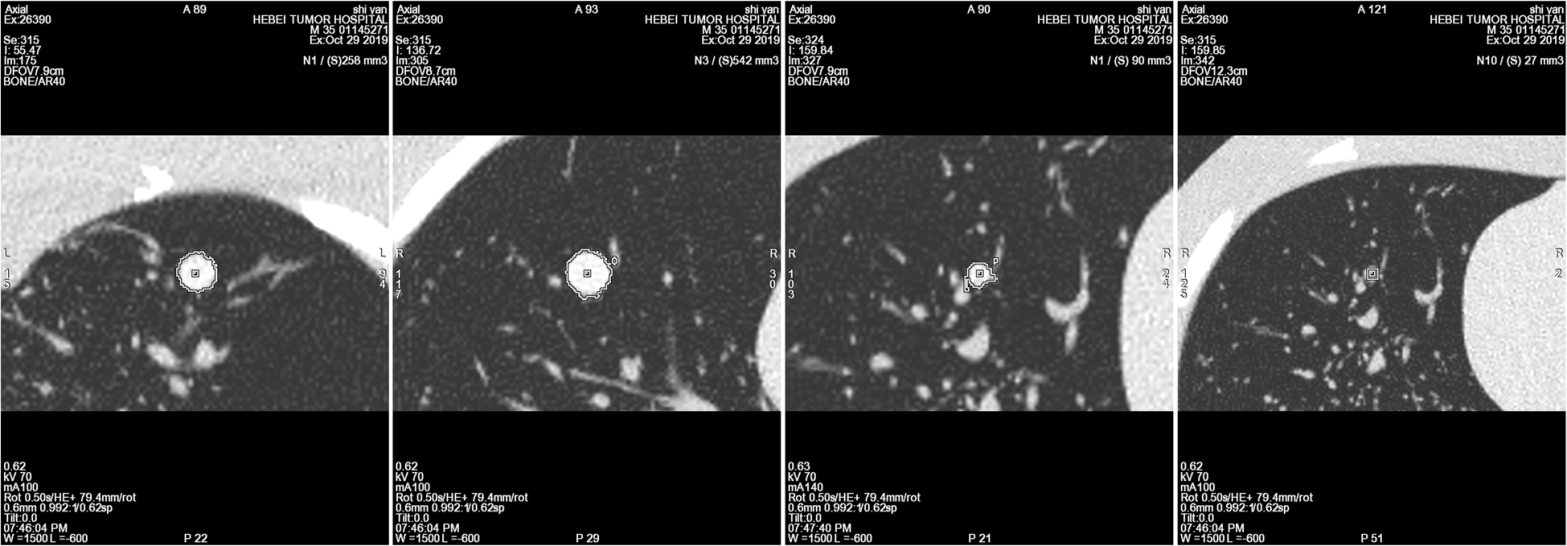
CT images of pulmonary nodules of different sizes
